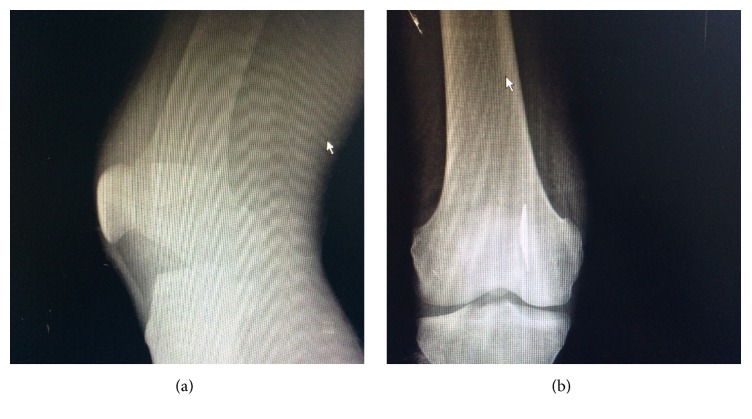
(a) Lateral radiographic examination of the knee. (b) Anteroposterior radiographic examination of the knee.
